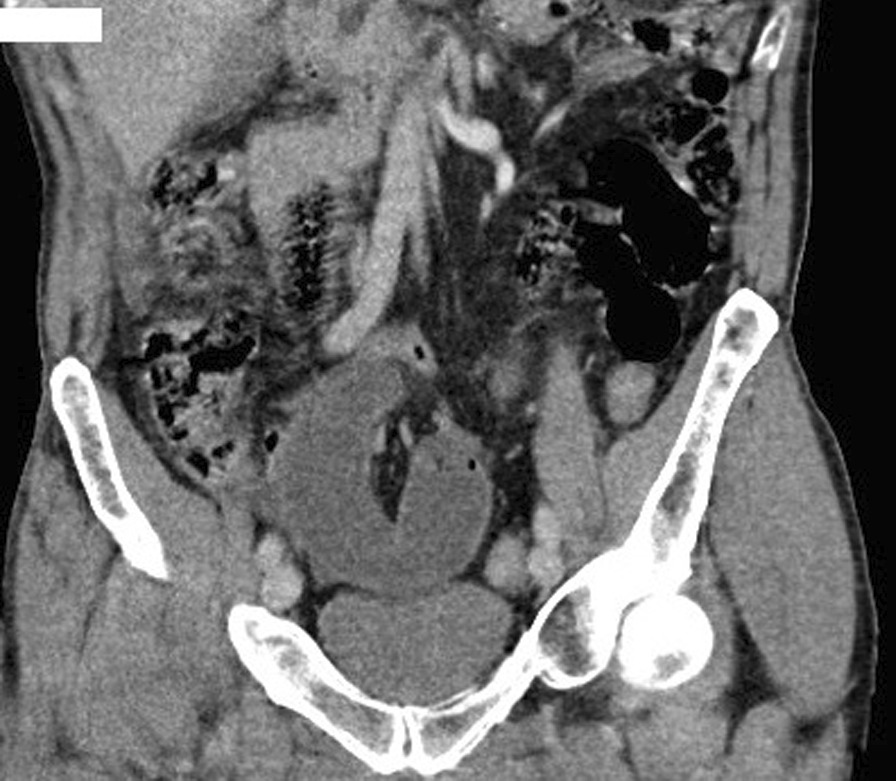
CT scan. White line refers Meckel's diverticulum
